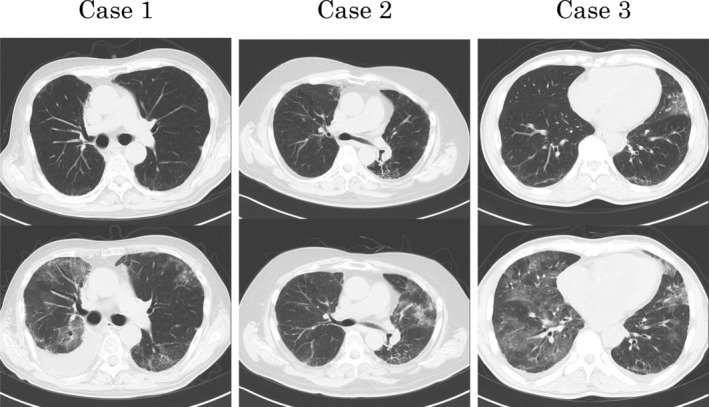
Thoracic computed tomography of the three patients. Compared with the images of the upper row obtained before the onset of interstitial lung disease (ILD), images of the lower row show diffuse ground‐glass opacities in all cases at the onset of ILD.
